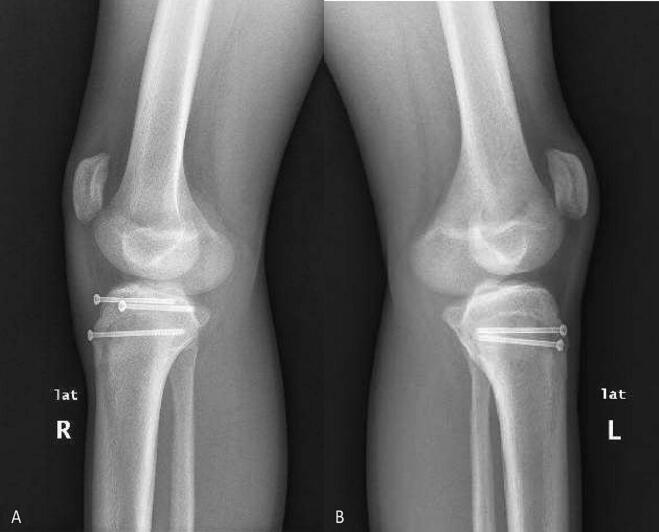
Six-month postoperative lateral plain radiographs of both knees. (A) Right knee showing three 4.0 mm partially threaded cannulated screws. (B) Left knee showing two 4.0 mm partially threaded cannulated screws.
